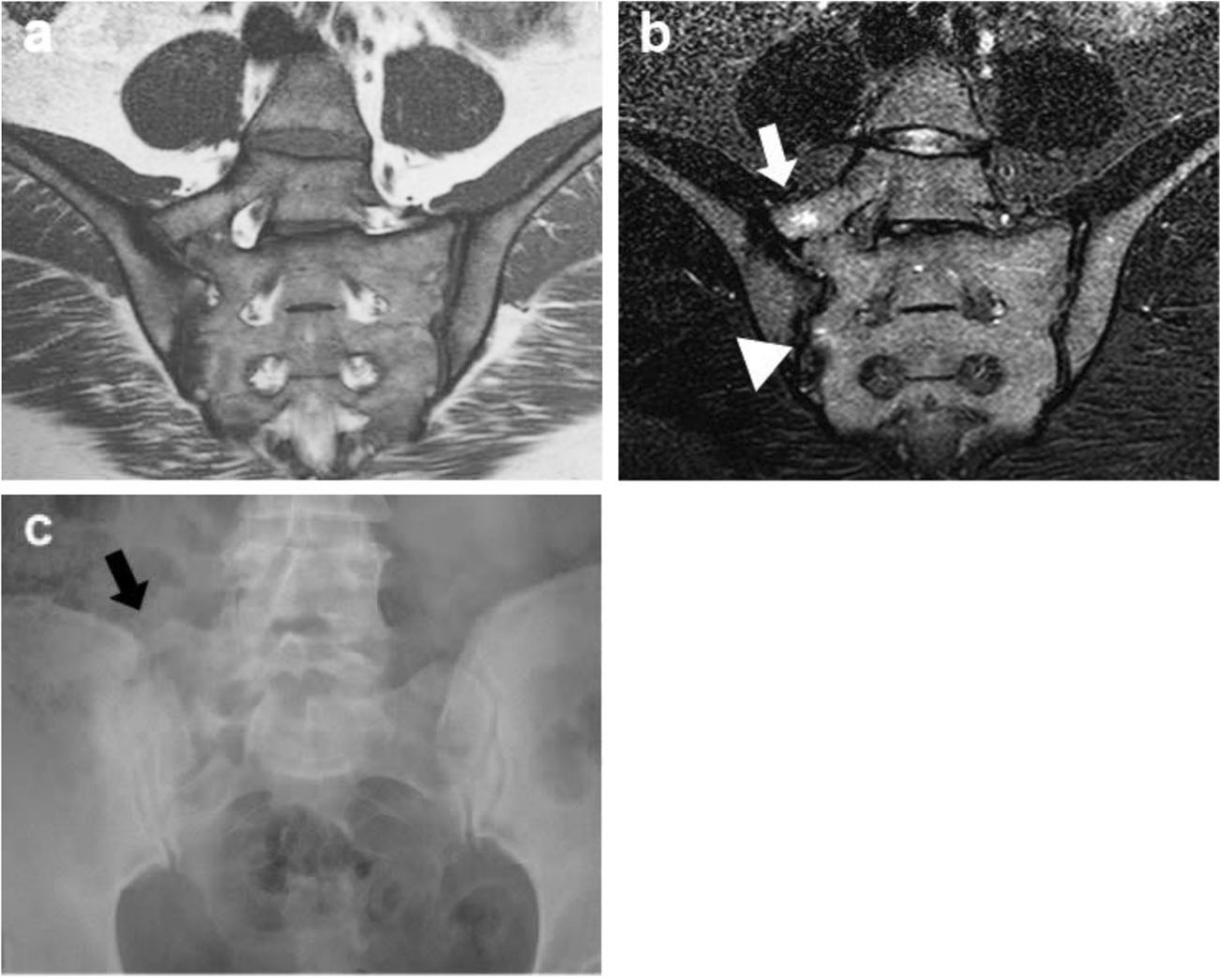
Example of a patient with local edema at the pseudoarticulation site. a T1-weighted, b STIR and c anteroposterior radiograph of the sacroiliac joints in a 38-year-old woman with axial spondyloarthritis. a Pseudoarticulation. b Bone marrow edema is visible at the site of the pseudoarticulation (*white arrow*), without reaching the sacroiliac joint. In addition, subtle bone marrow is visible in the lower half of the sacroiliac joint (*arrowhead*). Although the bone marrow edema in the right SI joint is subtle and not sufficient to fulfill ASAS criteria, it demonstrates that bone marrow edema can occur in the two locations in one patient and that these two lesions are not likely to be confused. c Unilateral LSTV grade II (*black arrow*)
